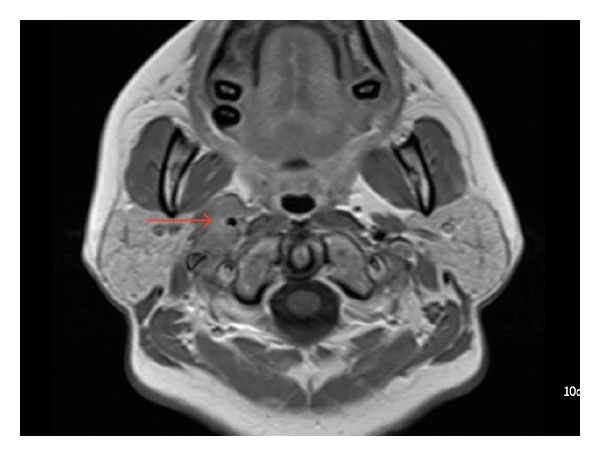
MRI scans of our patient showed multiple right-sided swollen lymph nodes. They were subsequently biopsied.
